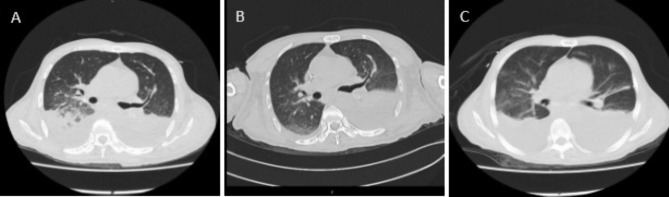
The chest computed tomography imaging during disease progression. Compared with the chest computed tomography on admission day and Day 10 (A and B), the inflammation on Day 17 progressed significantly (C)
